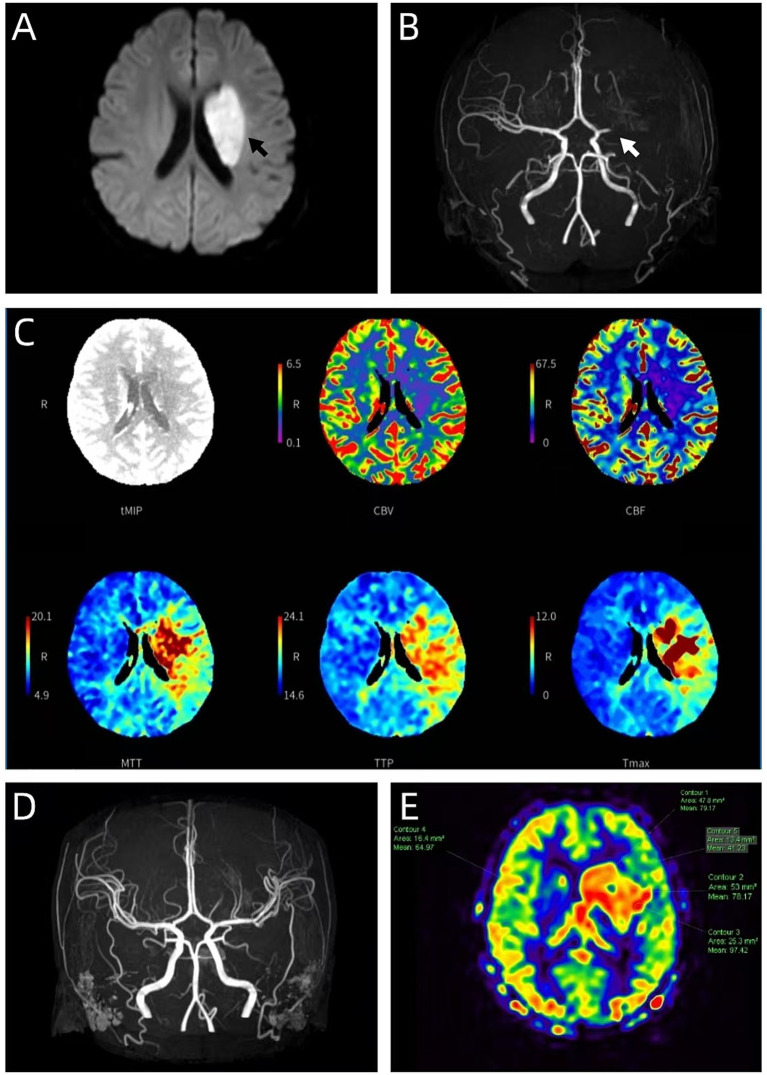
Neuroimaging findings during the initial acute phase and following treatment. (A) Brain DWI sequence shows a patchy area of hyperintensity in the left basal ganglia and periventricular region, consistent with acute cerebral infarction (arrow). (B) Brain MRA reveals focal luminal narrowing and significant stenosis of the left M1 segment of the middle cerebral artery (arrow), with poor visualization of the distal segment. (C) Brain CT perfusion (CTP) imaging demonstrates a large area of hypoperfusion within the left MCA territory (involving the frontal, parietal, and temporal lobes, basal ganglia, and insula). Quantitative analysis indicates a core infarct volume of 7.9 mL, a hypoperfusion volume of 66.5 mL, and a significant ischemic penumbra (mismatch volume 58.6 mL, mismatch ratio 8.4). (D) Follow-up MRA after treatment shows restoration of the lumen in the left M1 segment, with a normal caliber and course, and no significant stenosis. This dynamic evolution from stenosis to normality is consistent with recanalization following resolved cerebral vasospasm. (E) Repeat perfusion imaging shows significantly increased perfusion (hyperperfusion) around the original left basal ganglia infarction, consistent with post-ischemic hyperperfusion.
